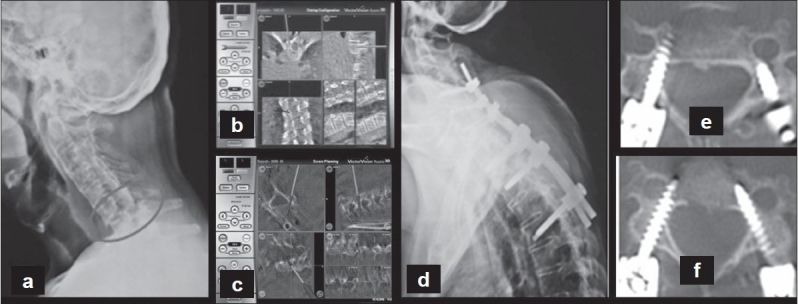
(a) Preoperative lateral radiograph of cervical spine showing a C6-7 chance fracture in a patient with ankylosing spondylitis (b, c) Intra operative navigation images of thoracic and cervical spine- pedicle entry point and trajectory and planning of screws (d, e, f) Postoperative radiograph and CT scan showing the placement of the screws
